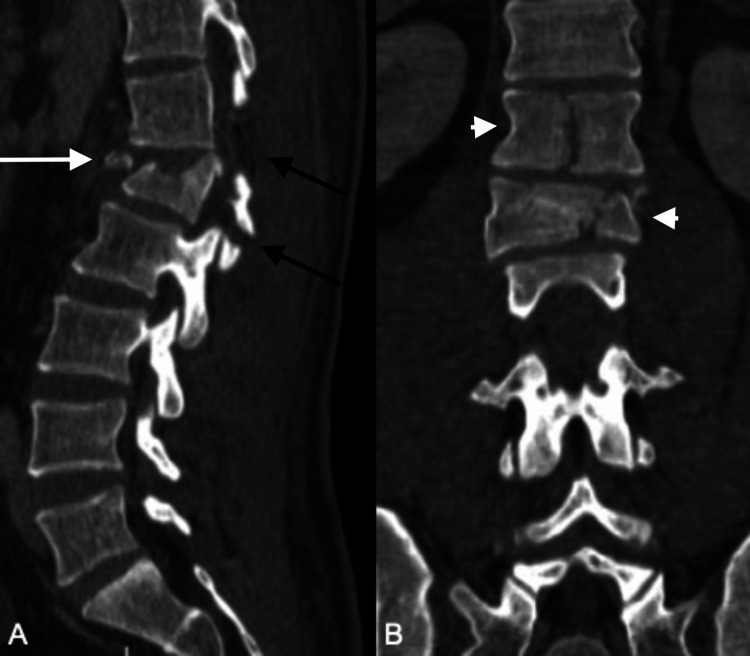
Preoperative sagittal (A) and coronal (B) CT of patient 3 highlights the osseoligamentous posterior tension band injury (AOSpine subtype B2) (black arrows) and significant complete burst fracture (AOSpine subtype A4) of L1 (white arrow). The complete burst fractures (AOSpine subtype A4) of T12 and L1 (white arrowheads) are also demonstrated on the coronal (B).
